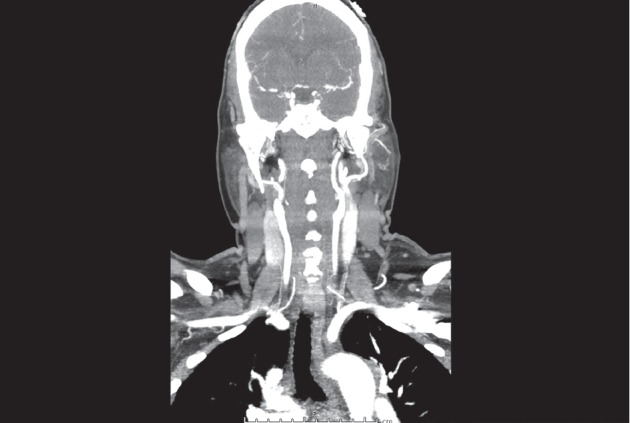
CT angiography shows patient blood circulation without tumor infiltration or encasement of the carotid body or the carotid sinus.
